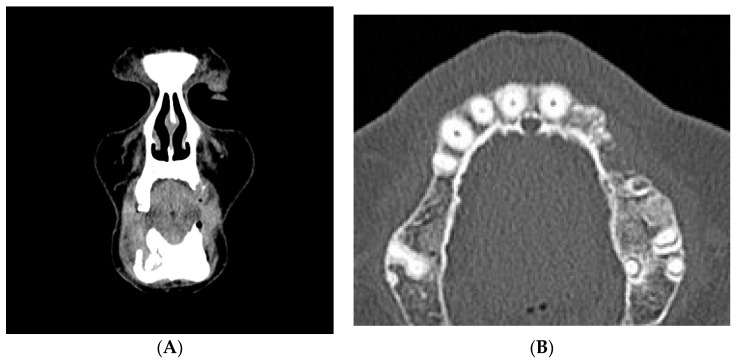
(A,B) CT scan dated February 2015. Coronal and axial cuts showing erosion of the left maxillary alveolar bone.
